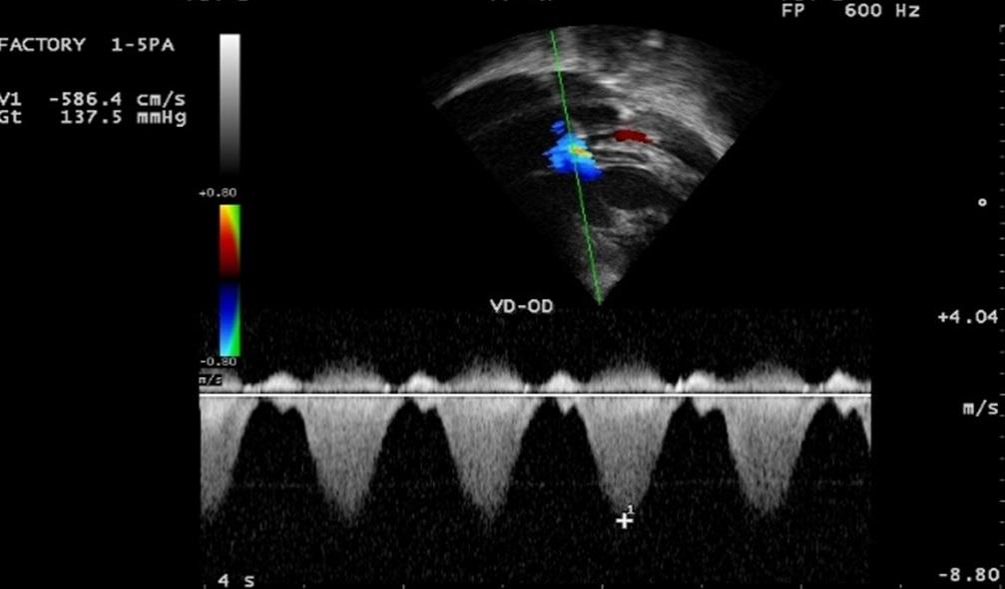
Pré-opératoire: Doppler continu sur le flux d'Insuffisance tricuspide (IT, en bleu); Gradient VD-OD de 137 mmHg
